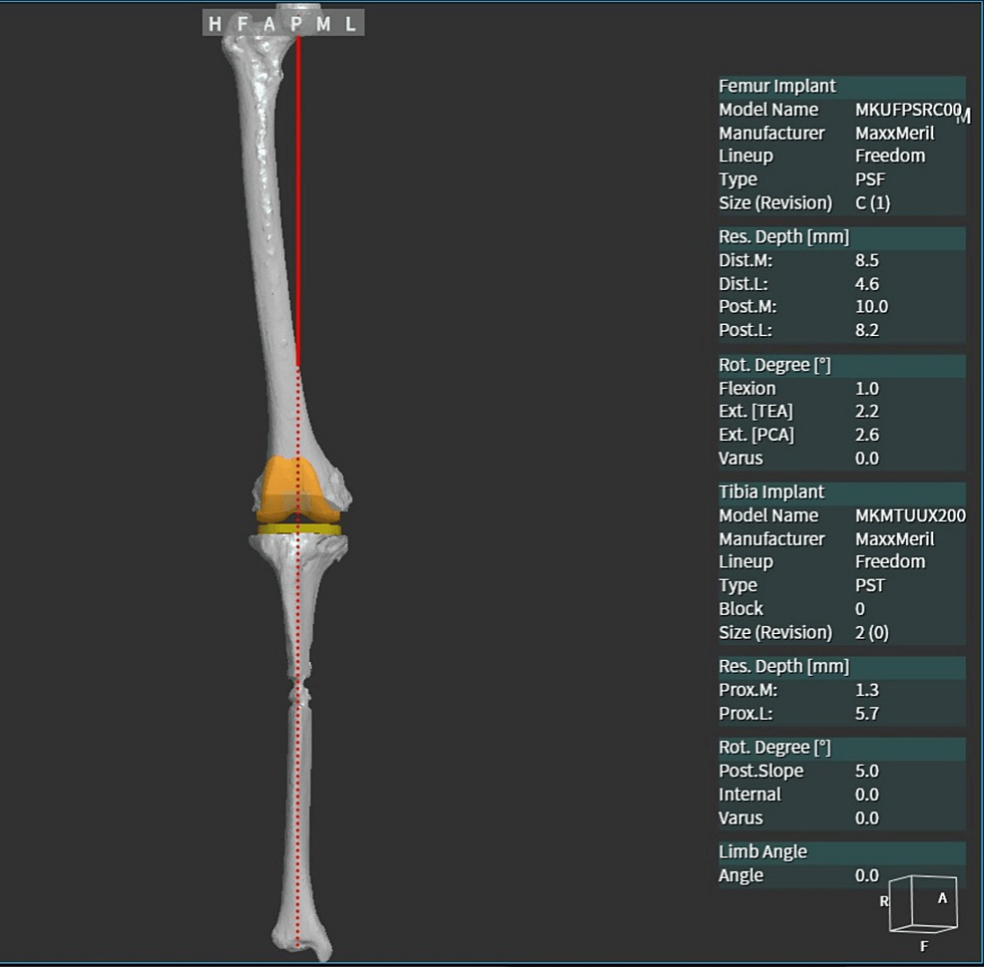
A representative image of the preoperative planning based on the preoperative 3D CT scan.
